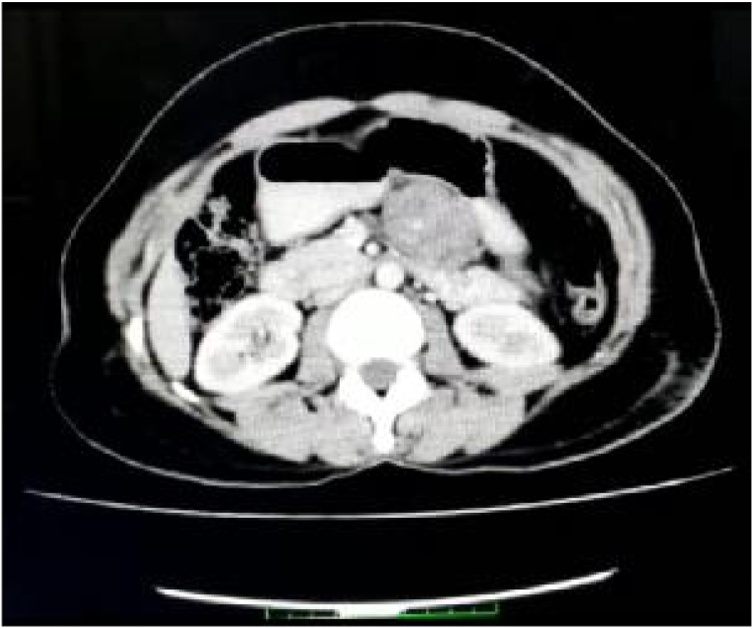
Contrast enhanced CT abdomen showing mass arising from body of pancreas with heteregenous enhancement and central specks of calcification.
